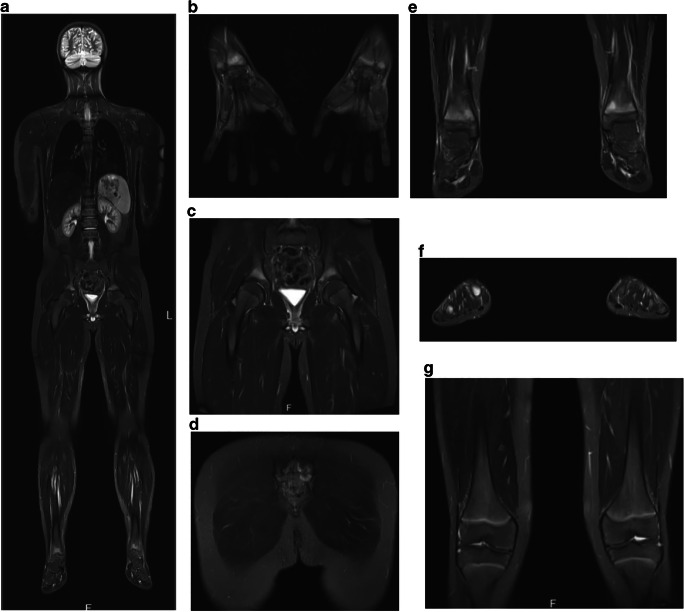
Whole body MRI of CNO. Whole body MRI using turbo inversion recovery measurement (TIRM) (a) of a 12-year-old male patient exhibiting symmetrical inflammatory bone lesions of the distal radius (b), distal tibiae (e), iliac bones (c), the sacral bone (d) and metatarsal bones (f). Knees were not affected (g)
